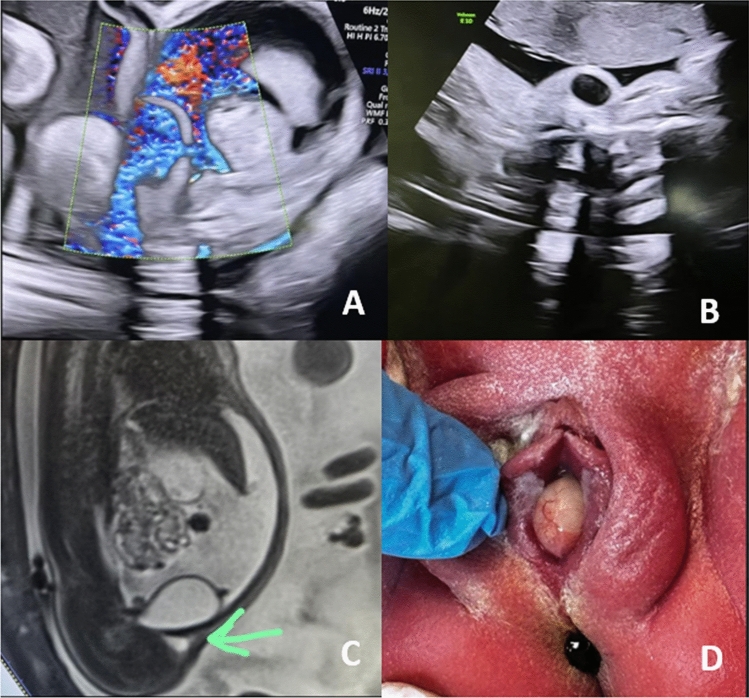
Prenatal and postnatal imaging of a paraurethral cyst complicated by bladder rupture. A Ultrasound with Doppler showing fluid flow from bladder and urinary ascites. B Cross-sectional view showing pelvic cyst lesion in the inguinal canal. C mid sagittal MRI view showing pelvic cyst lesion in the inguinal canal, D Postnatal appearance: cyst at vaginal introitus consistent with para ureteral cyst
